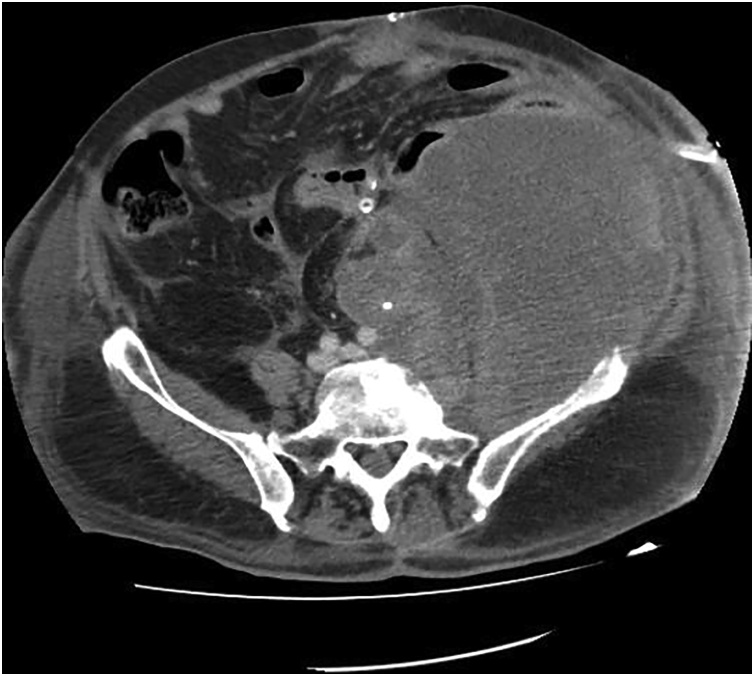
Postoperative CT showed rapid and massive primary tumor growth measuring approximately 20*14*15 cm in size with a 2.5-fold increase in volume.
